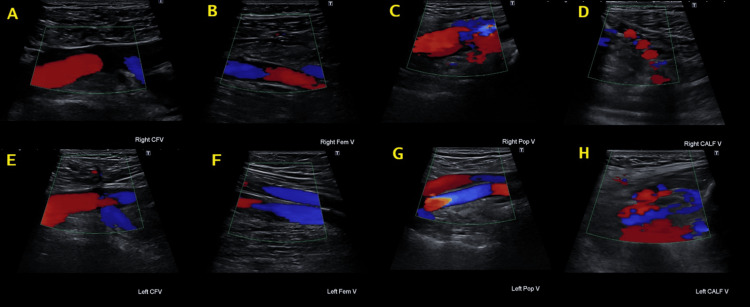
Ultrasound Doppler scan of lower limbs showing no evidence of thrombosis Ultrasound Doppler scans showing the right common femoral vein (A), right femoral vein (B), right popliteal vein (C), right calf vein (D), left common femoral vein (E), left femoral vein (F), left popliteal vein (G), and left calf vein (H). There is no evidence of thrombosis seen in any of the images CFV: common femoral vein
